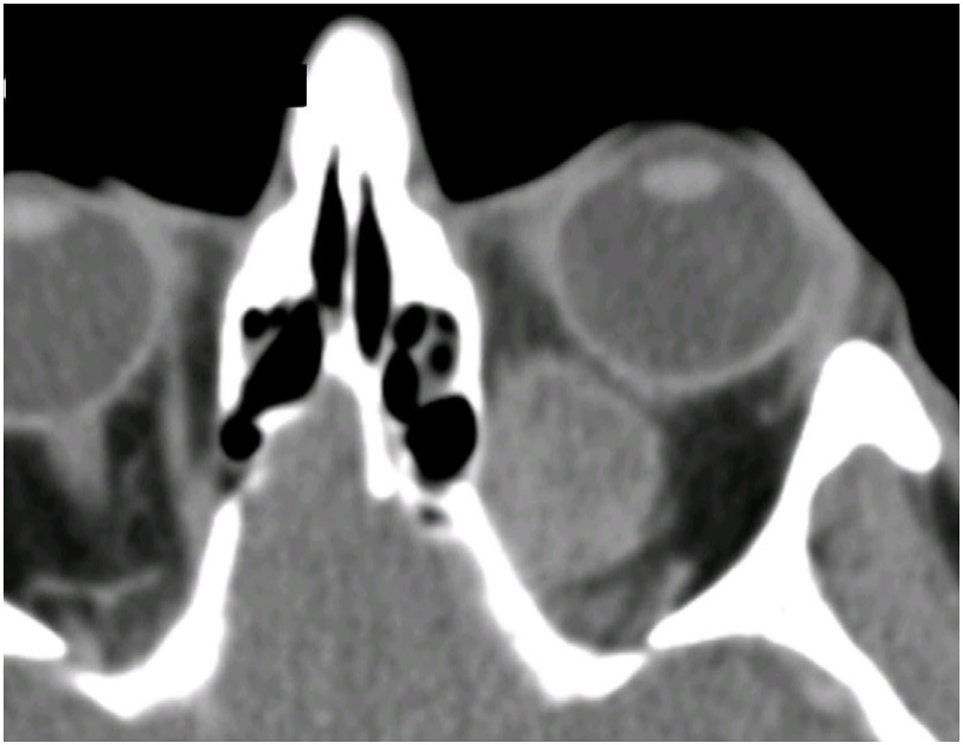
CT scan showing left orbital melanoma, with a typical configuration suggesting a well-defined benign mass; the rapid onset of symptoms over a few months, however, belies the sinister nature of the condition.
